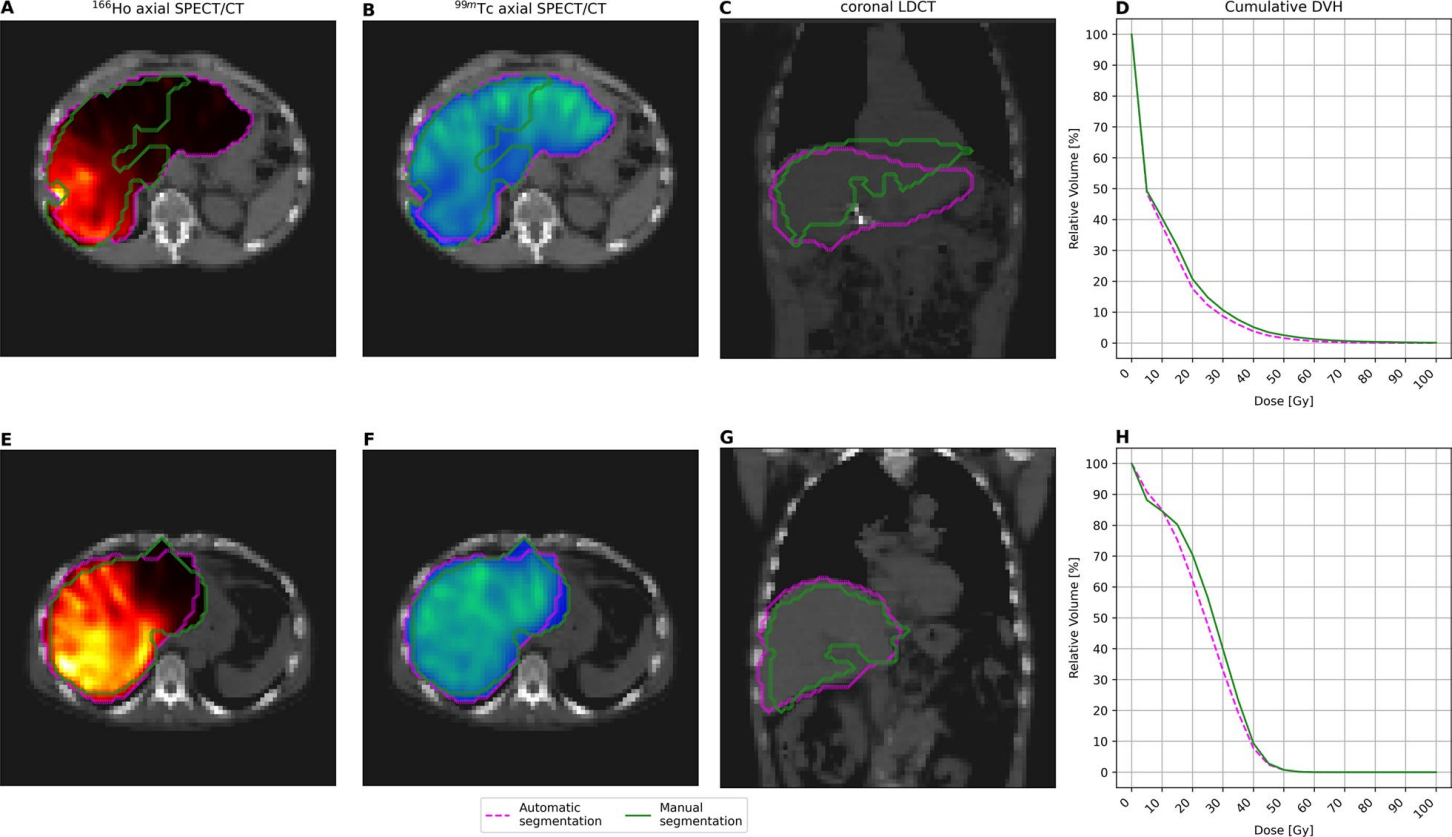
Examples of automatic segmentation outcome. A–D. A 56-year-old male subject treated with 3850 MBq of ^166^Ho microspheres in the right liver. E–H. A 65-year-old male subject treated with 4982 MBq of ^166^Ho microspheres in the right liver. Panels A and E, and B and F, show the axial view of ^166^Ho and ^99m^Tc SPECT/CT, respectively, while panels C and G show the coronal view of the LDCT acquired with the SPECT. Manual (solid green) and automatic (dashed magenta) segmentation is depicted. Upper panels (A–C, Sørensen–Dice coefficient = SDC = 0.62), show a clear discrepancy between segmentations, which is due to non-rigid deformation of liver because of breathing. Segmentations are quite comparable in the bottom panels (E–G, SDC = 0.84). Panels D and H show the resulting cumulative dose–volume histogram (DVH). Despite the segmentation differences, the corresponding dose difference is below 2 Gy
